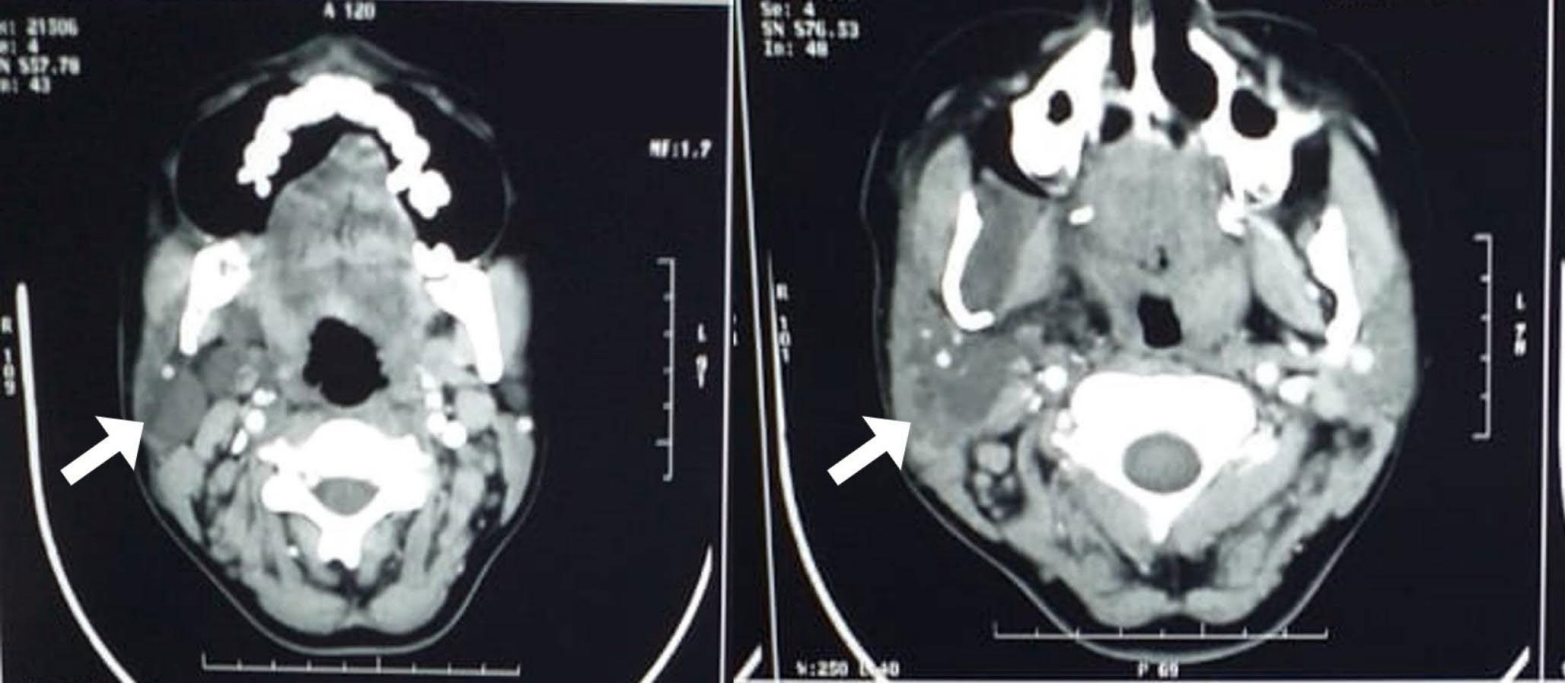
Contrast Enhanced Computed Tomography image of neck of lesion (white arrow)
